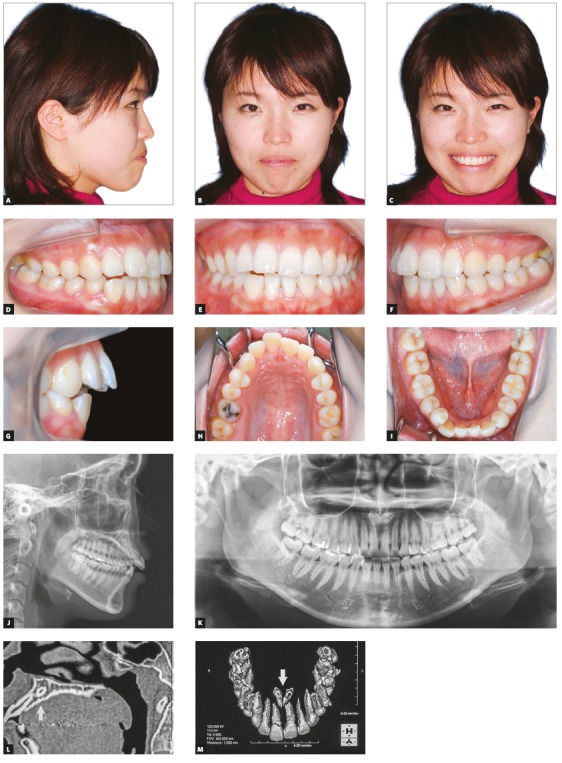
Pretreatment records (at 21 years and 3 months of age): A-I) extraoral and intraoral photographs; J) lateral cephalometric radiograph; K) panoramic radiograph; L-M) cone-bean computed tomography to assess the supernumerary teeth in the maxillary arch.
